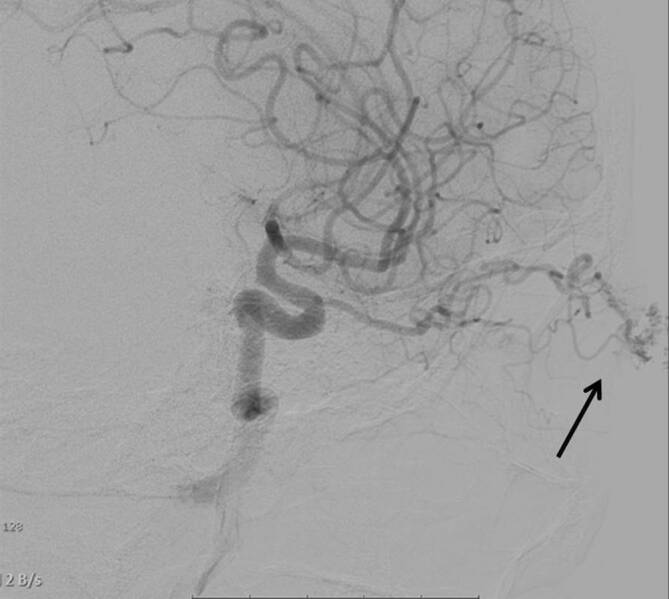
## Doppler- und Indocyaningrün-Angiographie-gestützte Resektion
